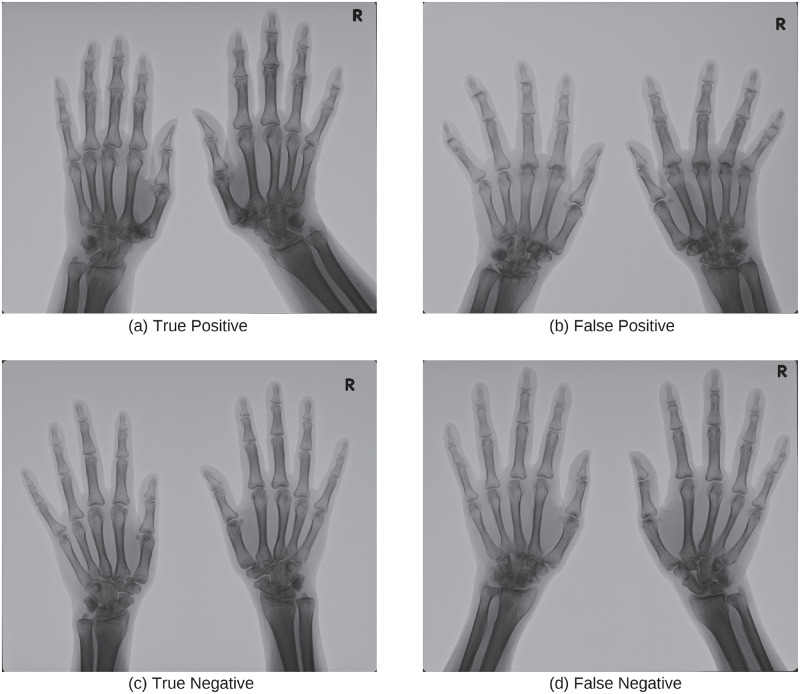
Examples of X-ray images in ankylosis dataset. The caption of each image indicates the prediction result.
